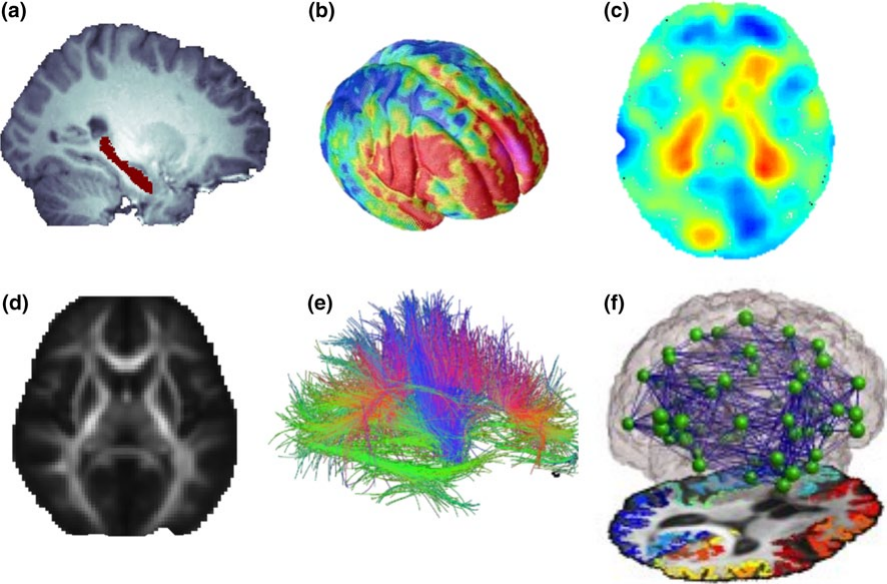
A variety of brain measures and features may be extracted from standard MRI and diffusion based MRI. When measured in a population of subjects, the observed variation in these brain traits can be studied in relation to genetic variation, and environmental influences such as education or diet, to identify factors that may promote or harm the brain. Population-based analyses include mapping out the overall degree of genetic influence or heritability for different brain measures, and effects of candidate gene or dietary or environmental factors. Features analyzed may include, but are not limited to: a volumes of brain structures such as the hippocampus; b cortical thickness or folding patterns; c regional brain volume differences with tensor based-morphometry maps; d maps of white matter integrity based on DTI, including measures of fiber coherence, such as fractional anisotropy (FA); e shapes and integrity of tracts of the brain; and f structural brain networks and the strength of cortical connections via white matter fibers, and topological measures based on such networks
